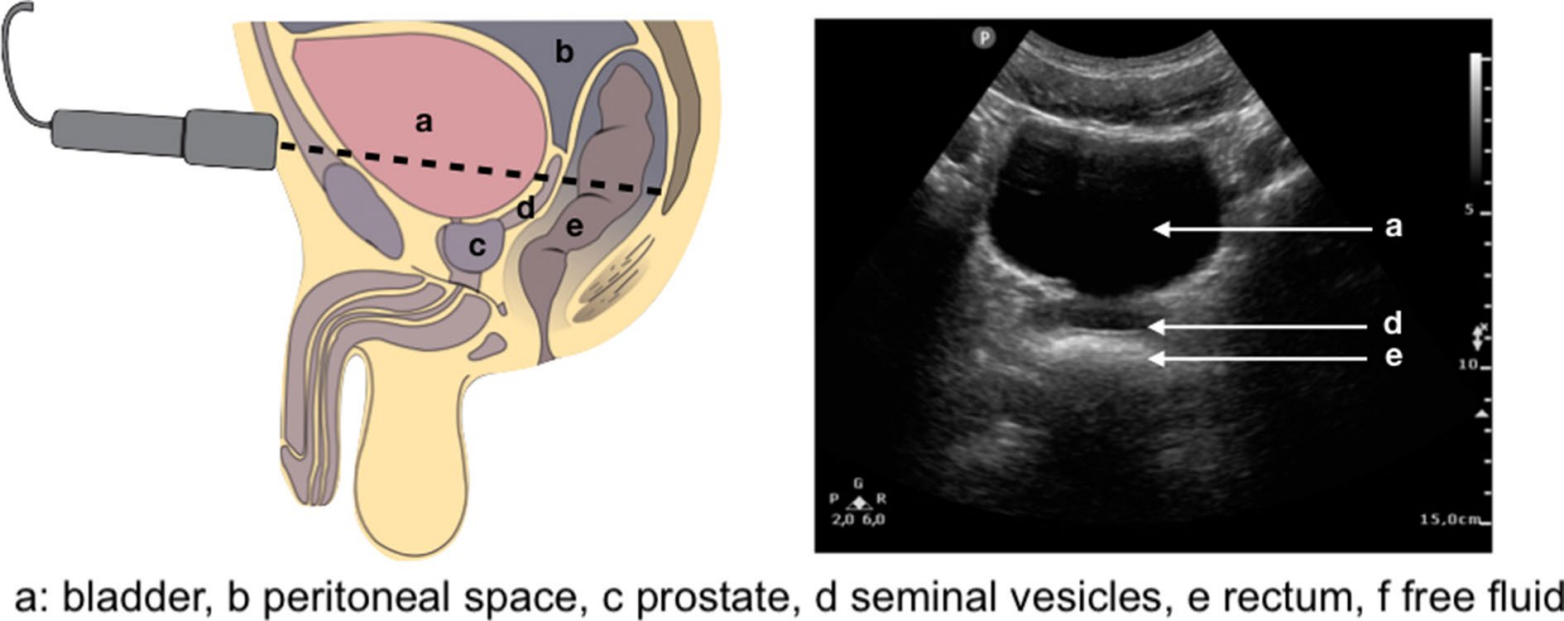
Transverse ultrasound view of the male pelvis—probe oriented towards the seminal vesicles
